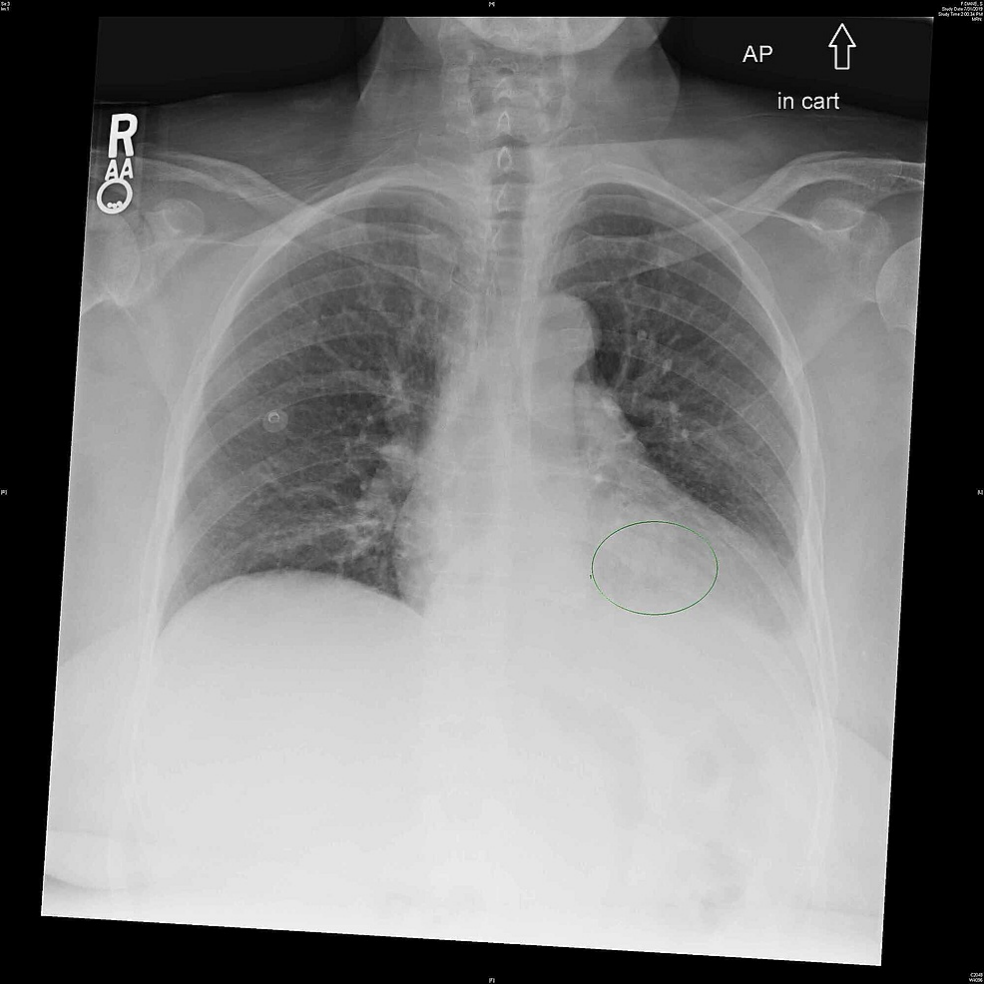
Chest radiograph was initially read as revealing a possible left lower lung opacity.
